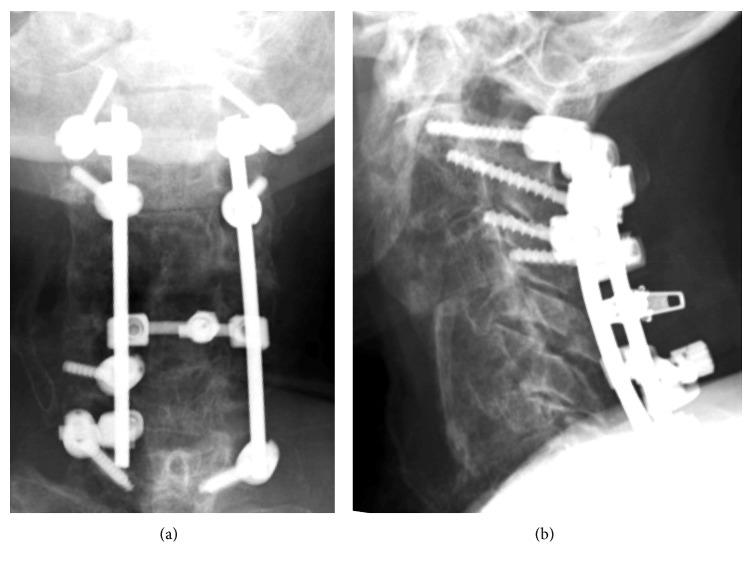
Plain radiography 1 year postoperatively. (a) AP view and (b) lateral view.
